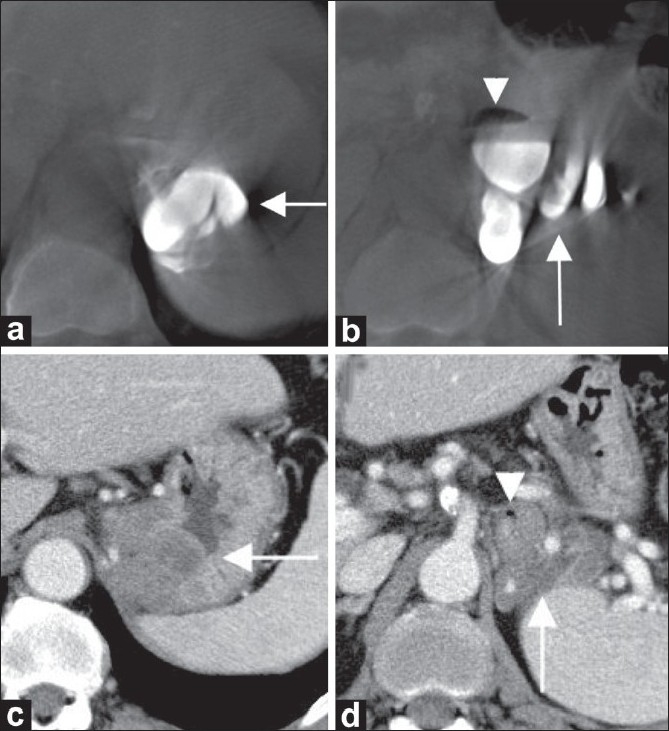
Unenhanced C-arm CT images (a,b) acquired just after the sclerosant injection and the corresponding contrast-enhanced MDCT images (c,d) obtained at 7 days after sclerotherapy to assess the sclerosant distribution. The transverse C-arm CT image (a) shows complete filling of the target varices (arrow) with sclerosant; this is confirmed by the corresponding axial CT scan (c), which demonstrates complete thrombosis of the target varices (arrow). The posterior gastric vein is entirely filled with the sclerosant (arrow in b), while the sclerosant is partially distributed to the gastrorenal shunt (arrowhead in b). Axial contrast-enhanced CT scan (d) also confirms that the posterior gastric vein is completely thrombosed (arrow), whereas the gastrorenal shunt remains patent (arrowhead). The sclerosant distribution seen in the unenhanced C-arm CT images coincides with that of the MDCT images
